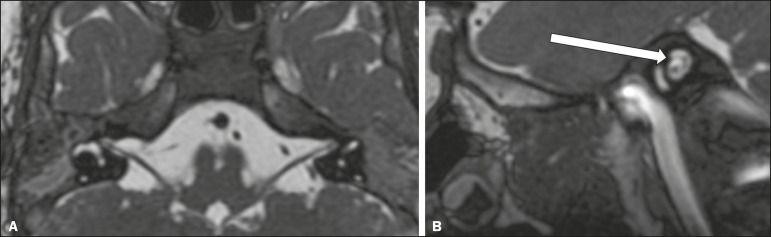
Moebius syndrome. Axial and sagittal 3D-SSFP MRI sequences (A and B, respectively) showing the absence of the facial nerve (arrow).
